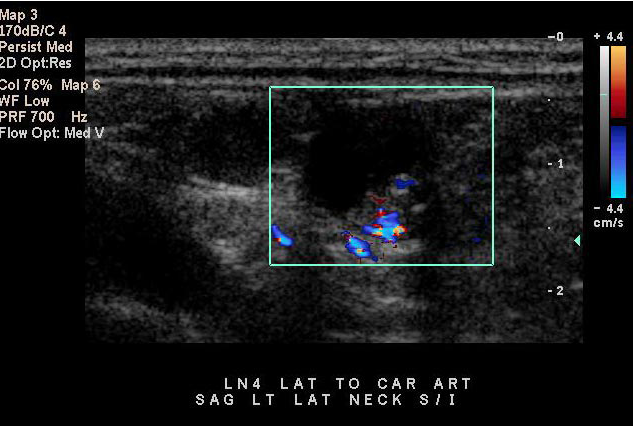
Ultrasound image demonstrates a mixed cystic and solid appearing lateral cervical lymph node in a patient with previous thyroidectomy for papillary thyroid carcinoma.
